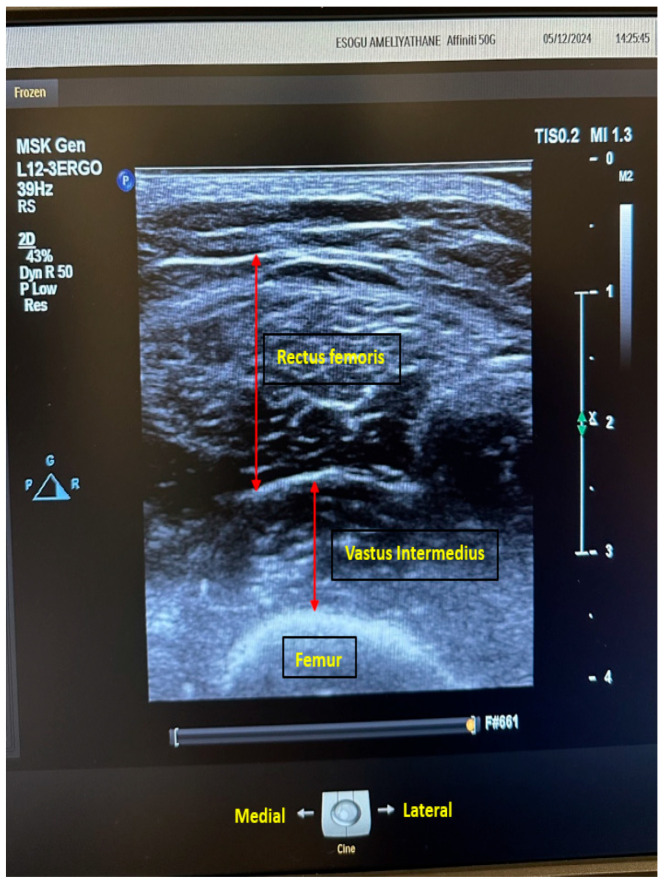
Ultrasonographic image of the thigh: measurement of rectus femoris and vastus intermedius muscle thicknesses (red arrows).
